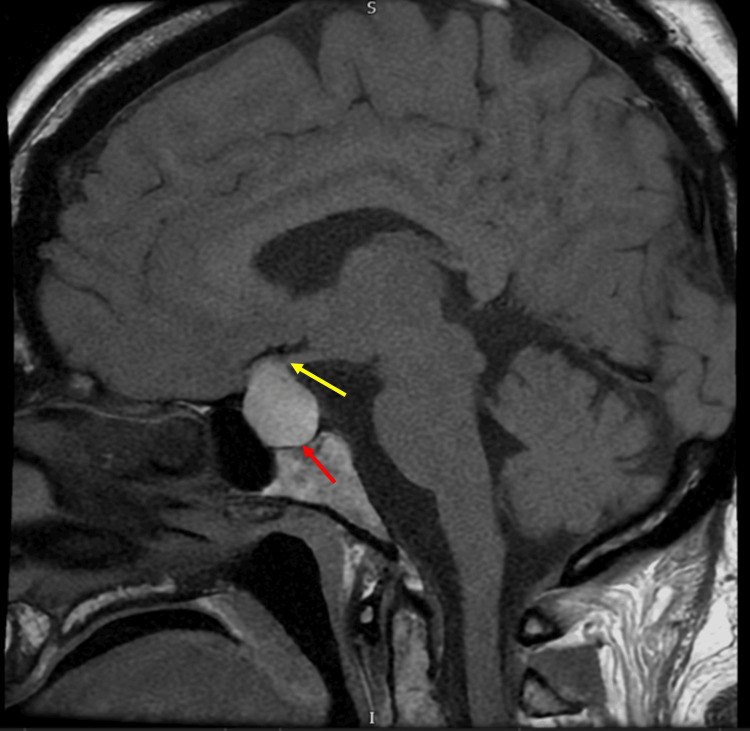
Contrast‑enhanced T1‑weighted sagittal MRI demonstrated a 21 × 13 mm sellar mass (red arrow) with high T1 signal intensity and no measurable contrast enhancement, showing suprasellar extension and compression of the optic chiasm (yellow arrow).
